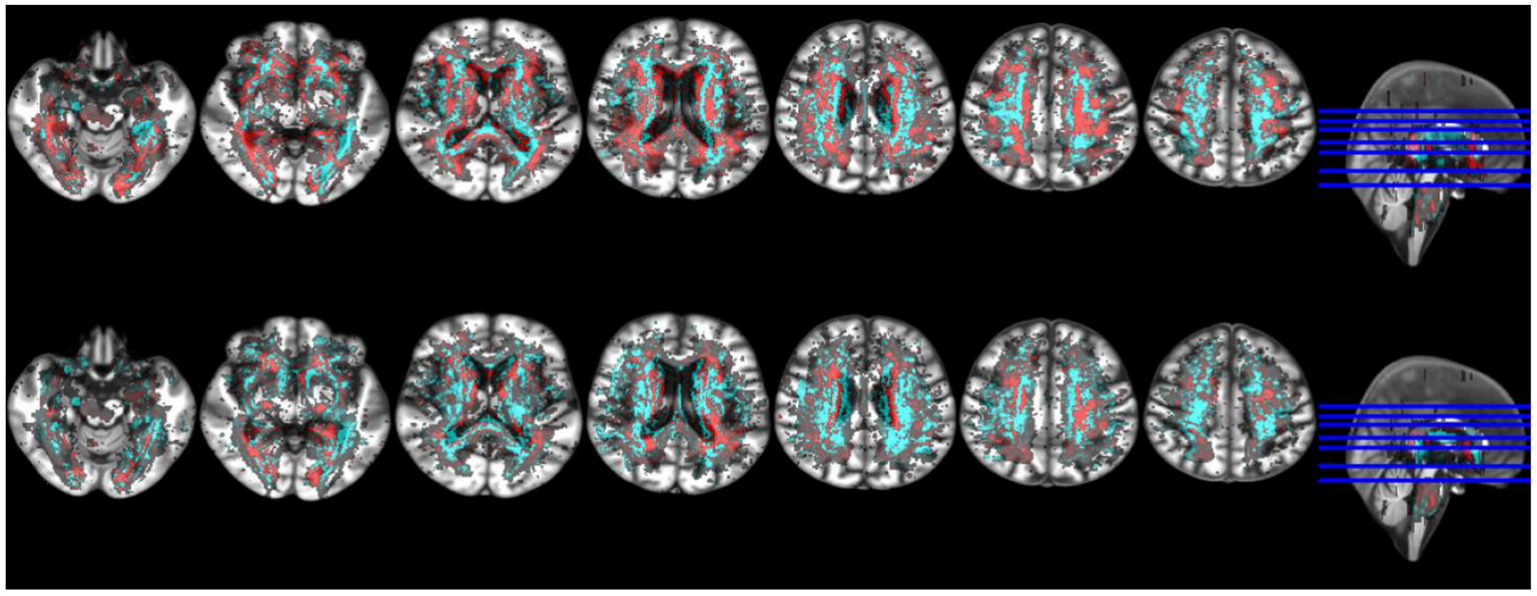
Selection of axial slices showing the probability distribution maps of the associations of WMH change with RSSI location of the primary (i.e., larger) DWI positive cluster accounting for age (top row) and RSSI location with baseline WMH (bottom row). In both, positive associations are shown in cyan and negative associations in red. Although positive associations were stronger than negatives in absolute values, maps were rescaled to the 50% of their maximum values to facilitate visual comparability.
